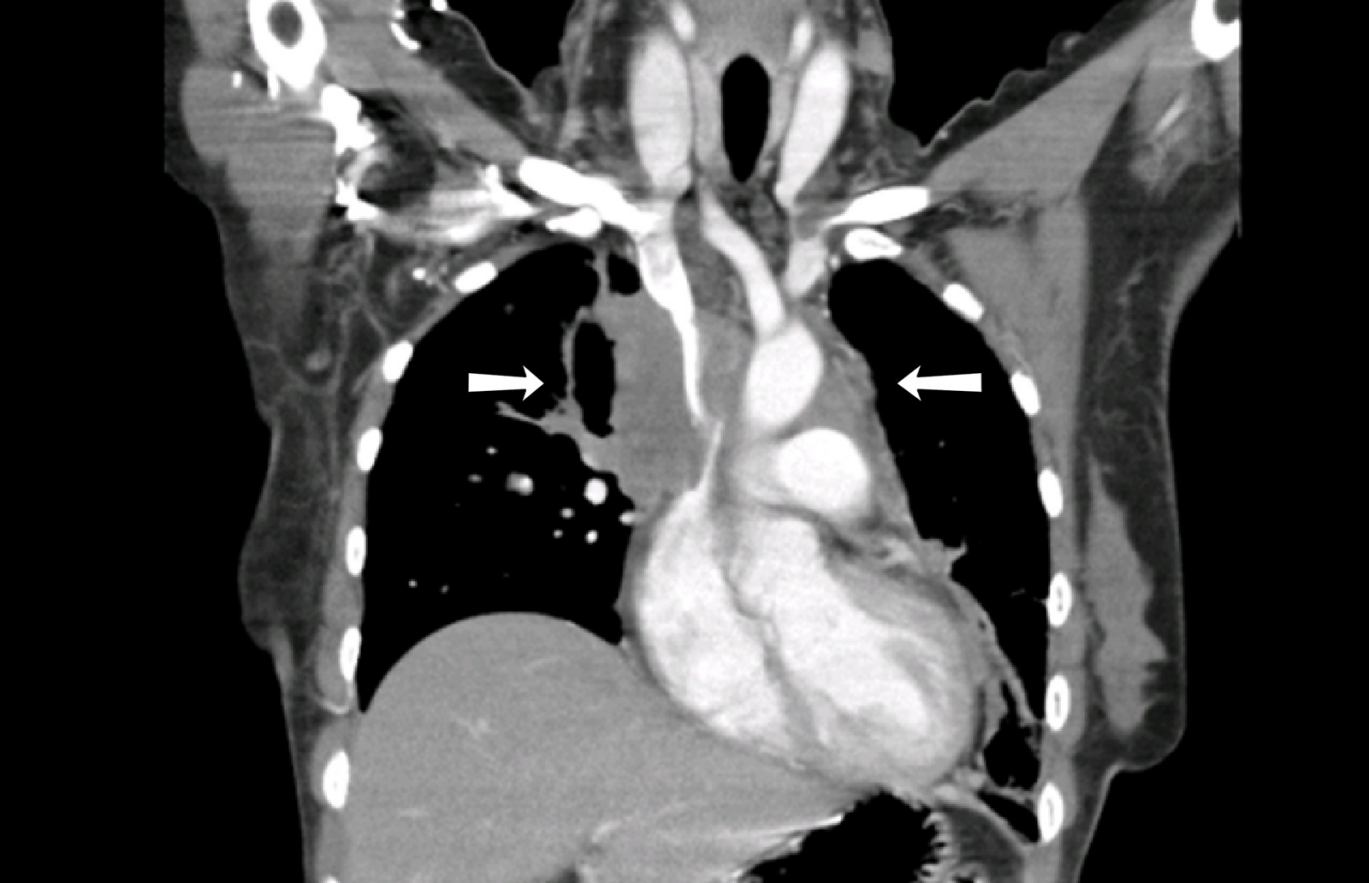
Image showing the computed tomography scan. A large ill-defined enhancing mediastinal soft tissue density mass encasing the heart and major vessels (white arrow).
